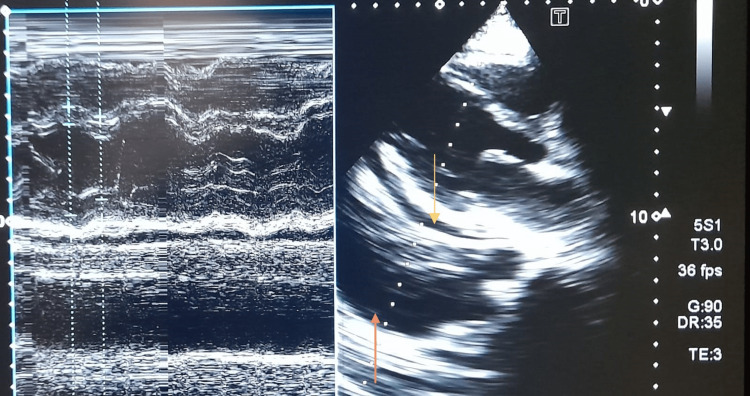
Two-dimensional echocardiography image (parasternal long axis view with M mode) showing mild pericardial effusion (yellow arrow) posteriorly and pleural effusion (orange arrow)
